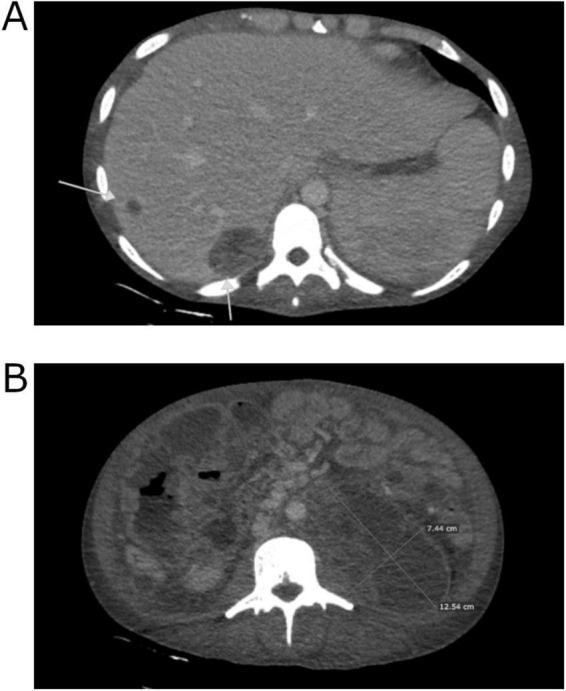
Computed tomography images of the patient’s abdomen from 28.01.2020 performed at The Maria Sklodowska-Curie National Research Institute of Oncology in Warsaw. (A) Regression of liver metastases. (B) Reduction in tumor size.
